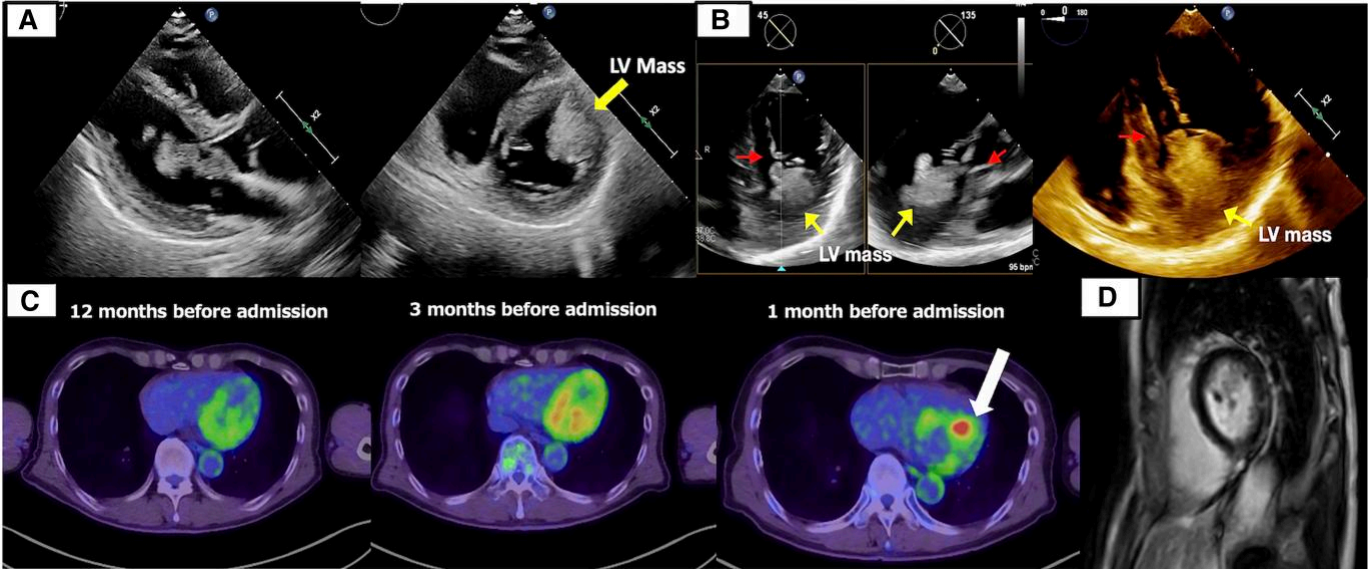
Multi-modal imaging of the left ventricular mass. (*A*) Transthoracic echocardiography revealed an irregularly shaped mass within the left ventricle. (*B*) Transoesophageal echocardiography demonstrated two cord-like structures measuring 20 and 19 mm (red arrows) extending from the mass. Part of the mass appeared to involve the left ventricular papillary muscles (yellow arrow). (*C*) Serial positron emission tomography–computed tomography images demonstrated increased metabolic activity in the left ventricle (SUVmax 7.12, white arrow) on the scan obtained 1 month prior to admission, compared with an earlier study, consistent with progressive tumour involvement. (*D*) Cardiac magnetic resonance imaging demonstrated no late gadolinium enhancement.
